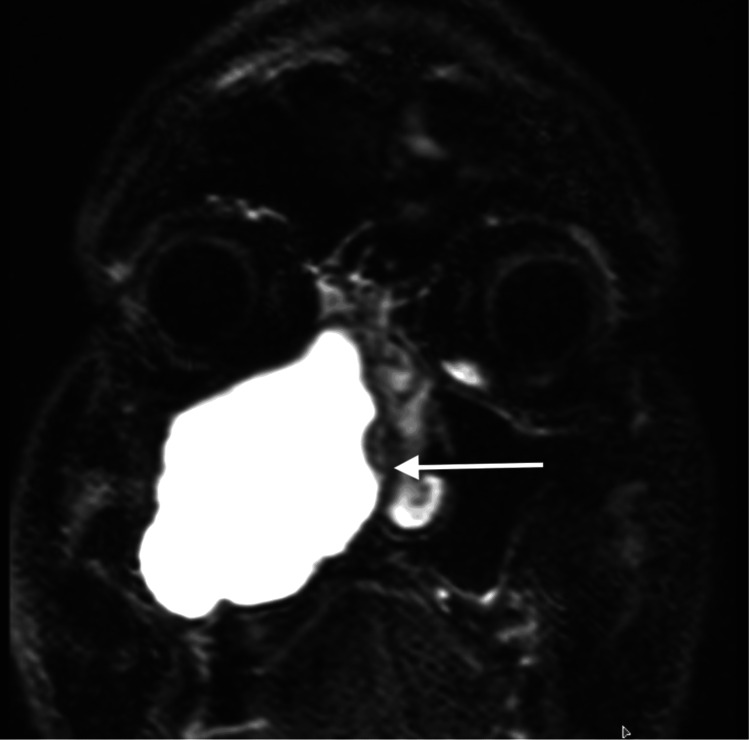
Coronal MRI of the paranasal sinuses Coronal T1-weighted MRI showing a well-defined expansile hyperintense lesion occupying the right maxillary sinus, with medial wall erosion and upward displacement of the orbital floor. MRI: magnetic resonance imaging
